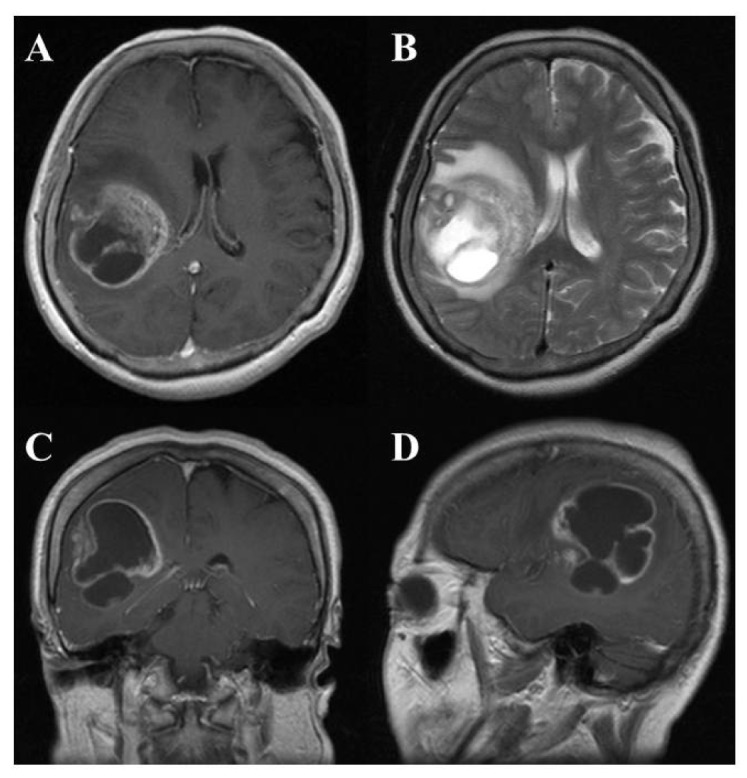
Representative radiological findings of GBM. Gadolinium-enhanced T1-weighted MRI (A, axial section; C, coronal section; D, sagittal section) shows a ring enhancement lesion in the right temporo-parietal lobe with midline shift. T2-weighted (B) image showed prominent peritumoral edema.
